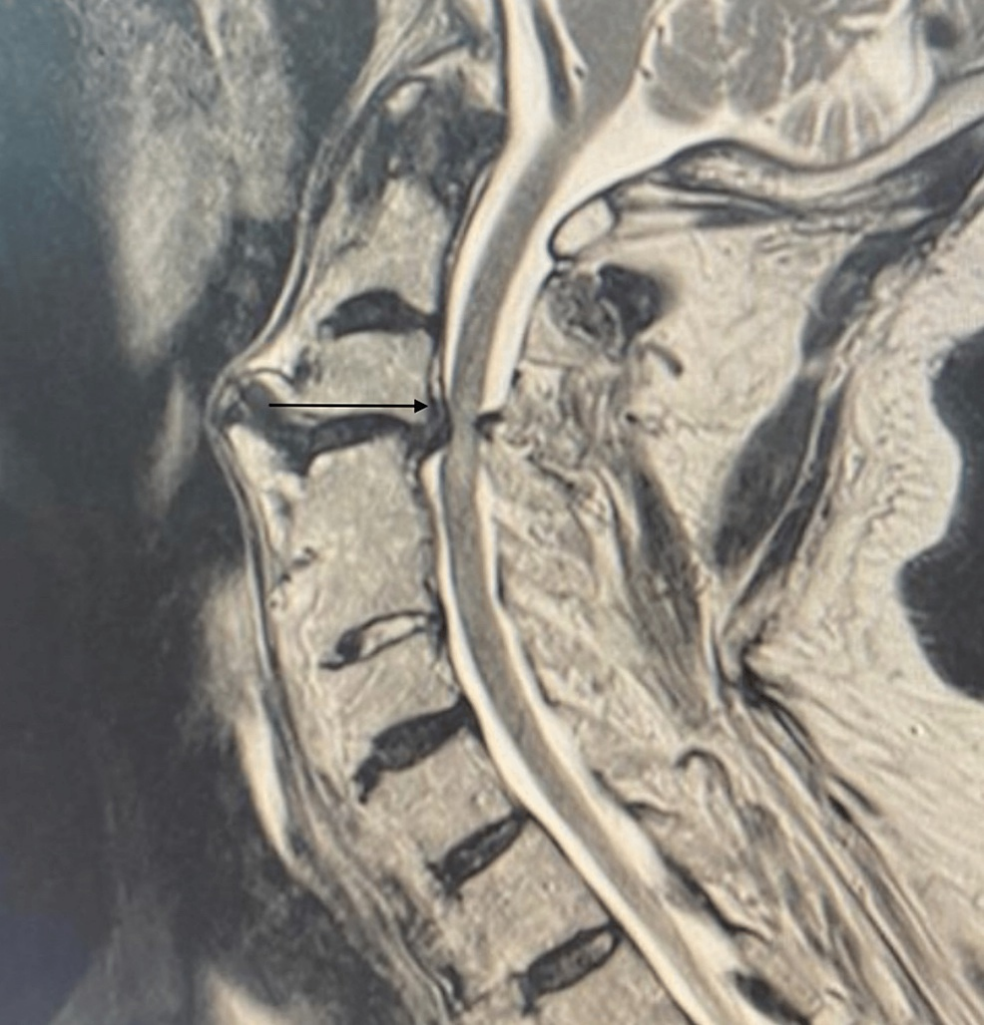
MRI cervical spine; sagittal view (T2) Showing a C3-4 cervical disc collapse compressing the spinal cord
